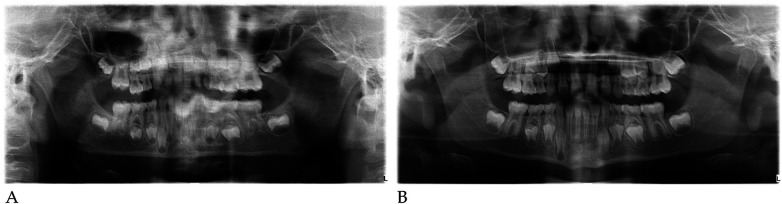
Pre- (A) and post-treatment (B) panoramic radiographs of case 1.
